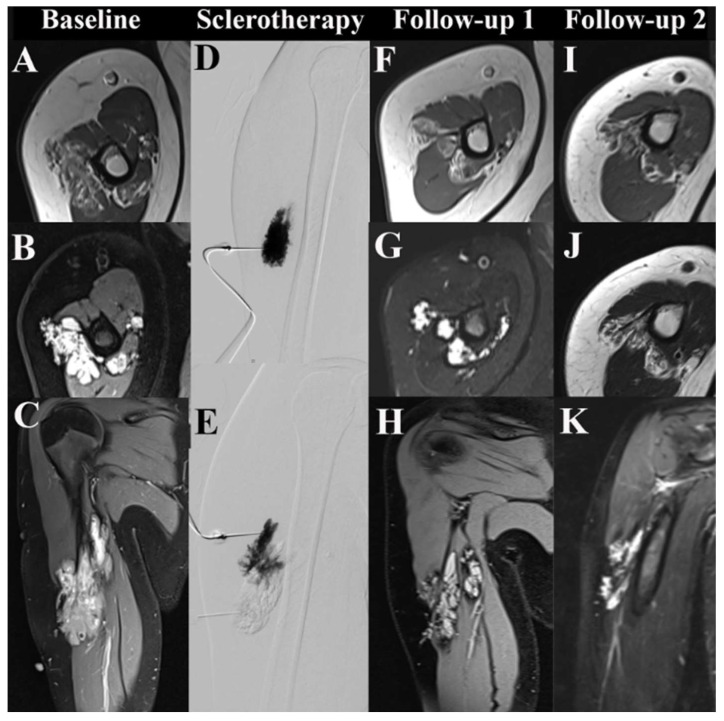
MRI of a low-flow venous malformation (VM) in the right upper arm before and after sclerotherapy. From top to bottom, axial T1-weighted sequences, axial T2-weighted sequences, and parasagittal contrast-enhanced sequences are shown in the first, second, and third rows. (A–C) show the baseline MRI of an untreated VM. (D,E) depict fluoroscopic images from ultrasound-guided percutaneous sclerotherapy using two direct punctures. (F–H) show MRI scans from follow-up 1 at seven months post-sclerotherapy. (I–K) depict MRI scans from follow-up 2 of the VM at 3.6 years post-sclerotherapy.
